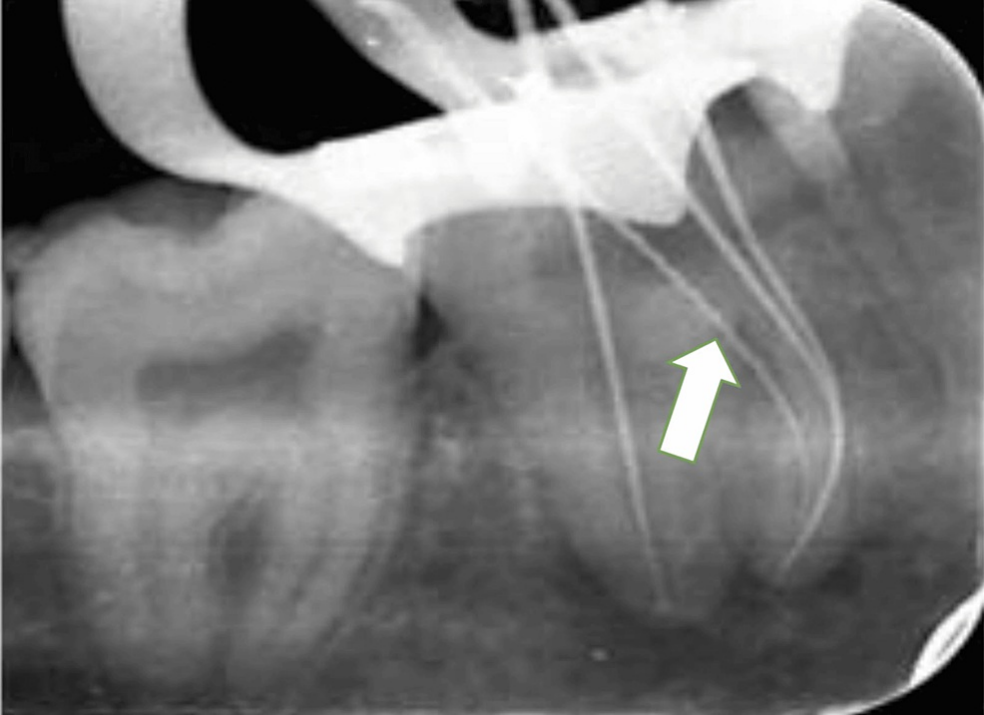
Working length radiograph Image by Dr. Khyati Manik
